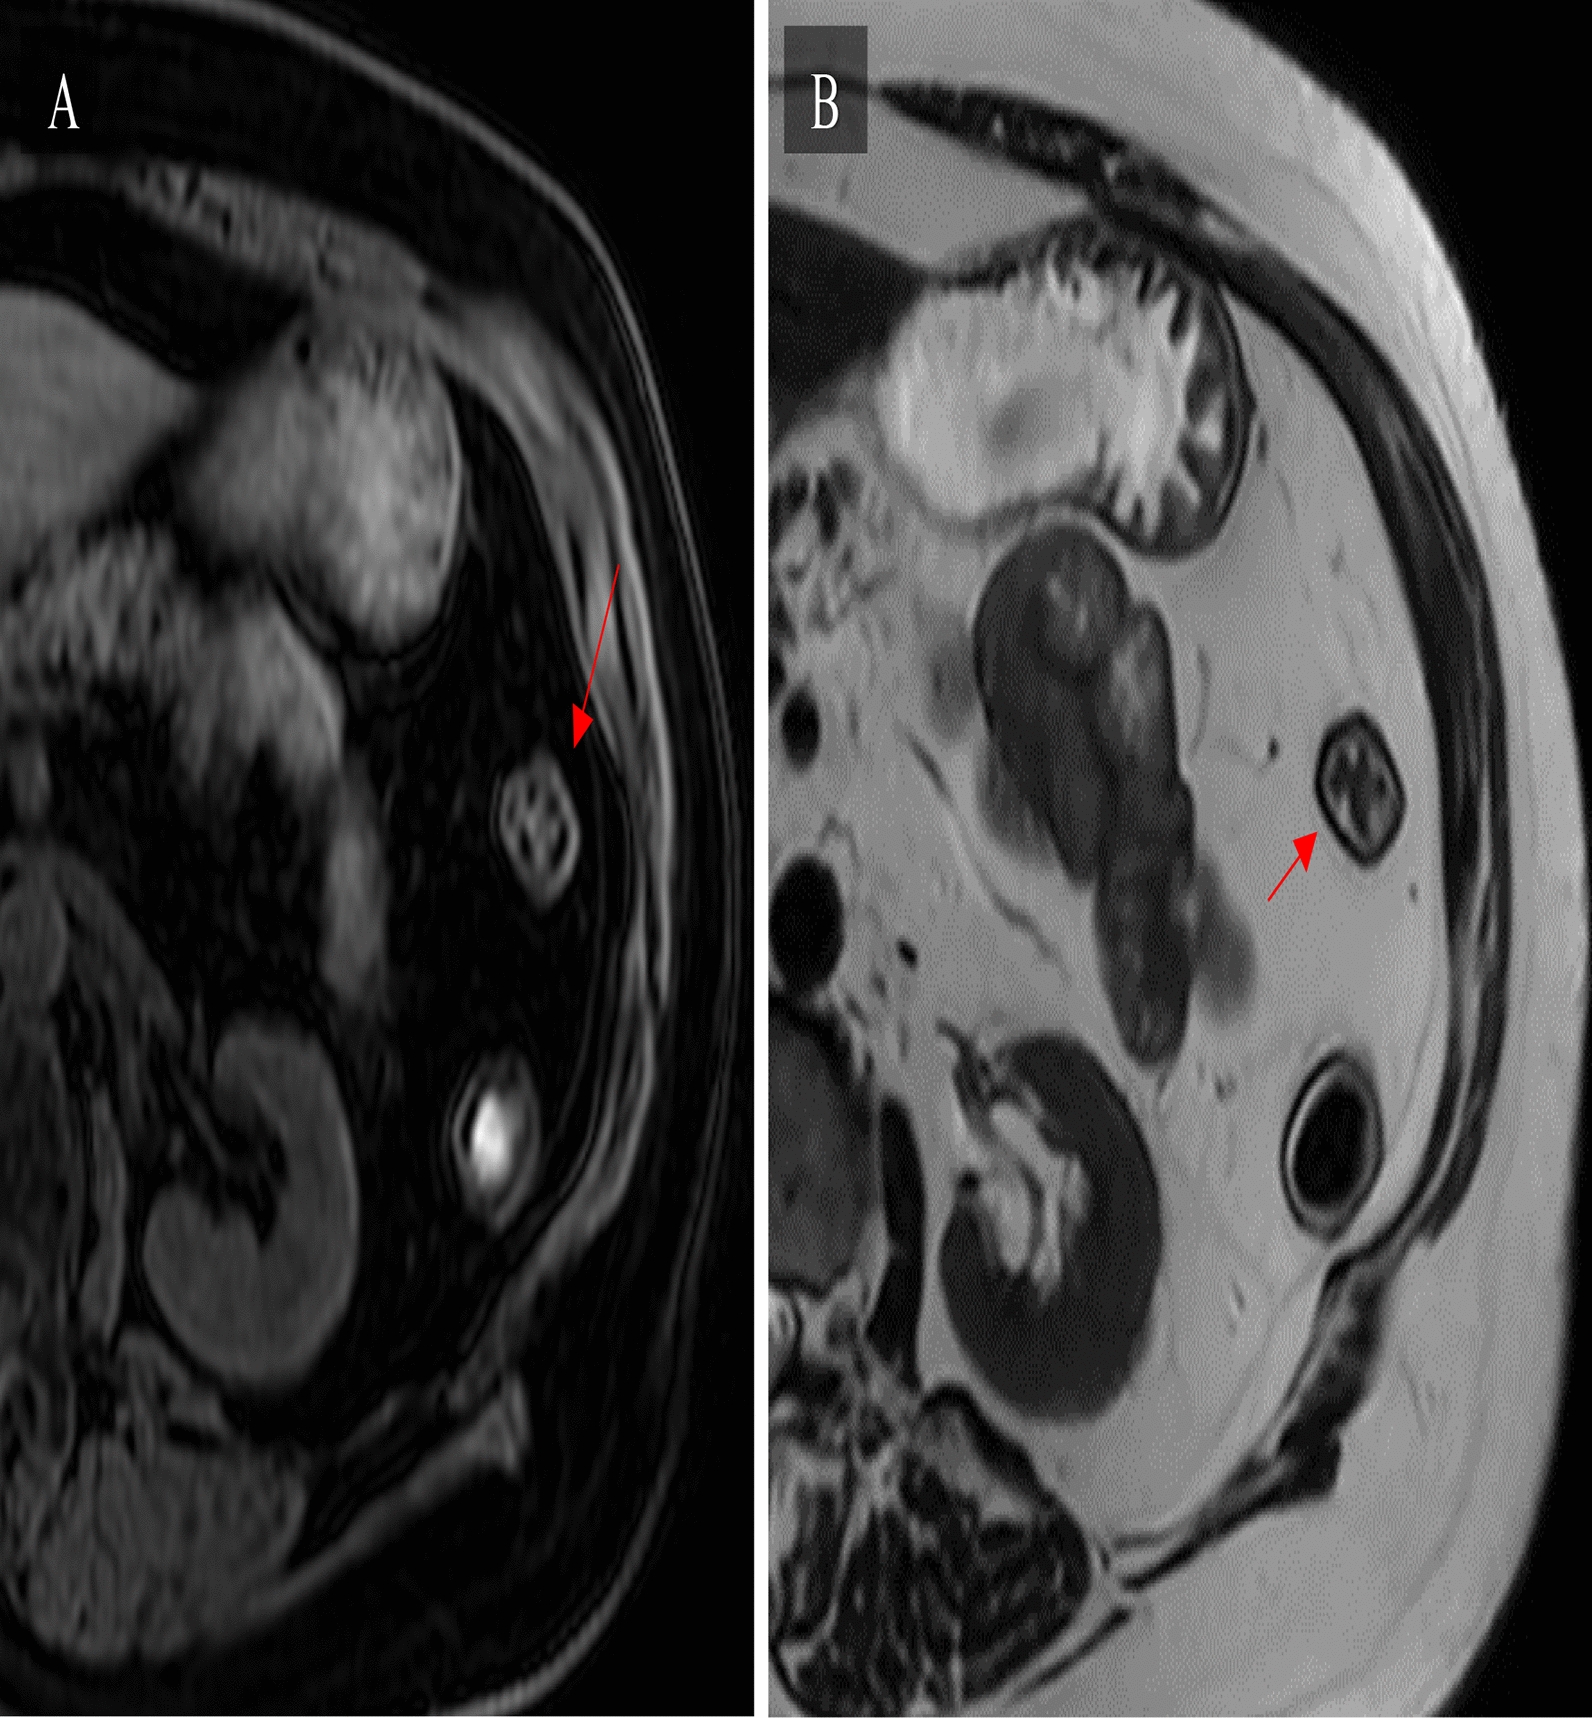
Axial T1 (A) and T2 weighted MRI (B) images highlighting submucosal fat deposition as well as thickened walls. See dark fibrotic wall on T2 (red arrow)
